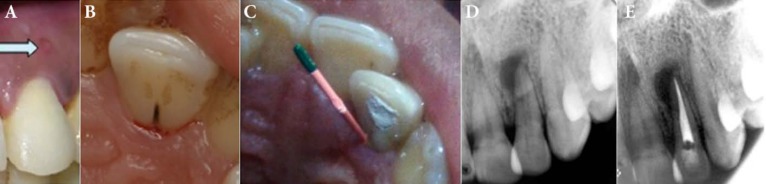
A)* Pretreatment clinical image of the maxillary left lateral incisor with sinus tract; *B)* Palatal groove; *C)* Tracing of the radicular groove; *D)* Periradicular lucency in pretreatment radiography; *E)* Post-endodontic radiography
